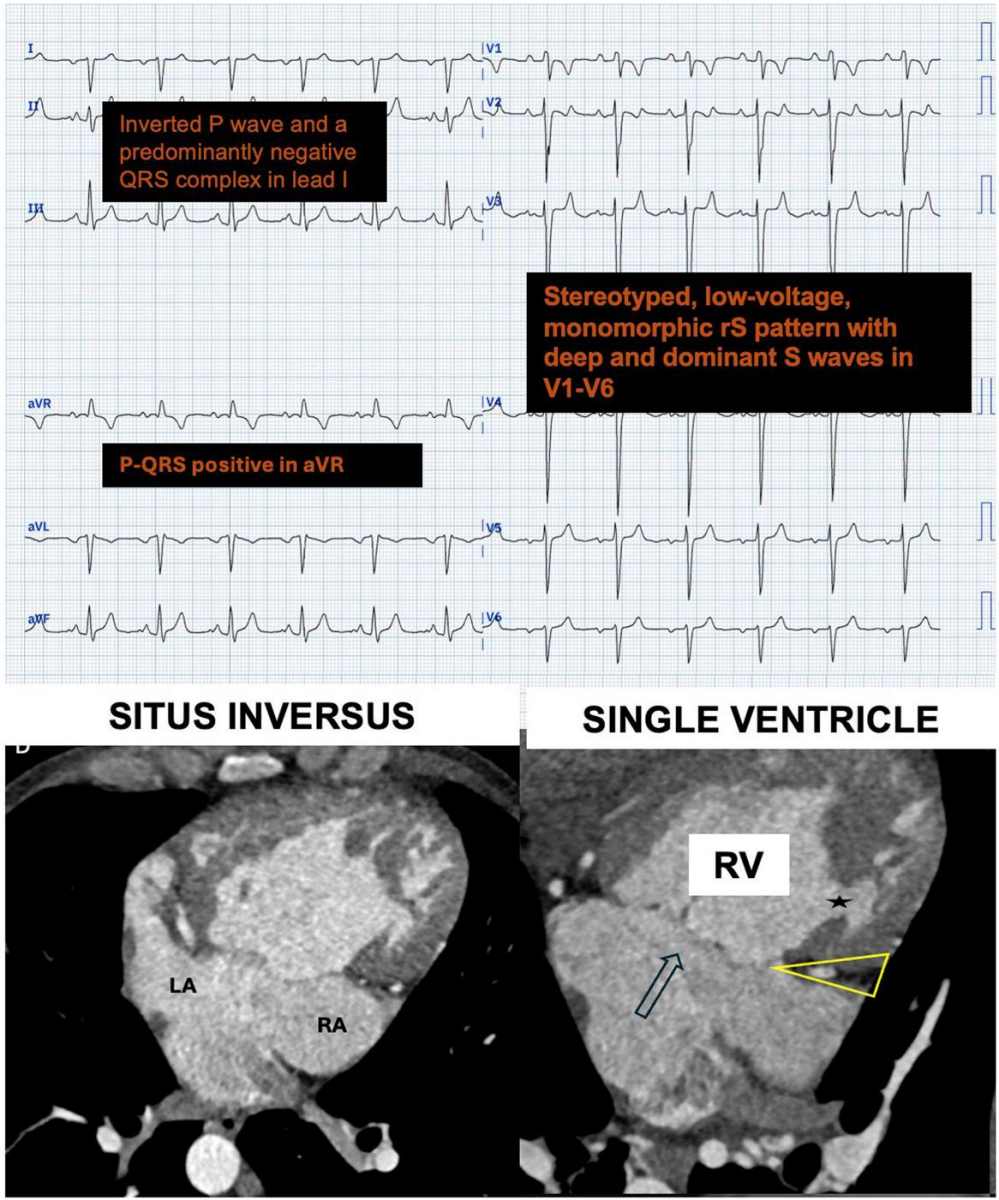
(Supplementary): CT multiplanar reformat images. *A*) Situs inversus totalis with Levocardia with levoapex, common atria and pulmonary veins draining into the LA. *B*) LA connected with a dominan RV (arrow), absent left AV connection (mitral atresia); thick muscular floor and left AV groove between the RA and hypoplastic LV (yellow triangle) and posterior hypoplastic LV and muscular VSD (star). Abbreviations: LA, left atrium; RA, right atrium; RV, right ventricle; LV, left ventricle; AV, atrio-ventricular; CT, computed tomography; SVD, single ventricle defect.
